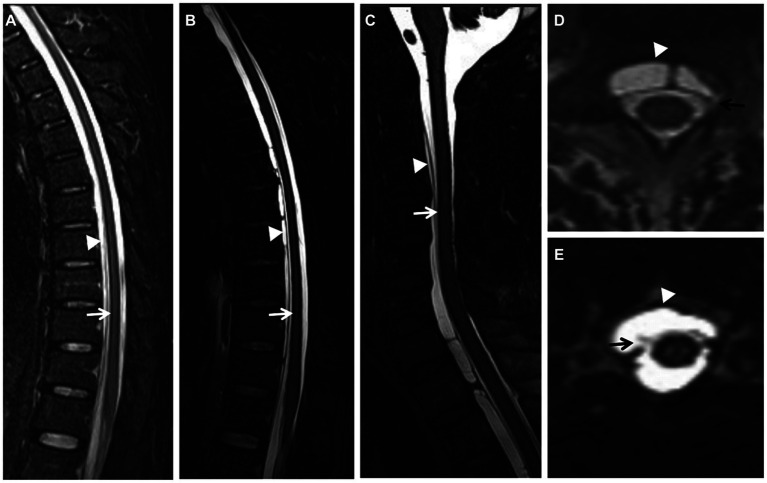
(Case 5) Extensive spinal superficial siderosis (SS) (A–C; arrows) and ventral epidural fluid collection at the cervical and thoracic levels (A–E; arrowheads). SS and ventral epidural fluid were more evident on T2-space sequences (B) than on T2-weighted images (WI) (A). Dural tear at the upper cervical level on axial T2-space sequence (D, E; arrows).
